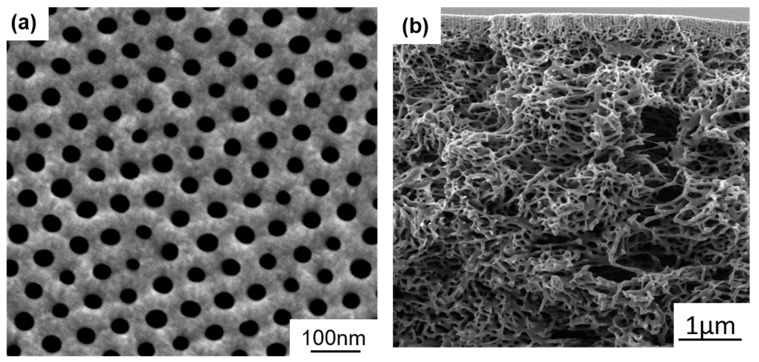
SEM images of (a) top view and (b) cross-section of PS_71_-*b*-P4VP_26_-*b*-PSMA_3_^145^ membranes cast from 23 wt. % solution in a THF/DMF/acetone: 50/30/20 wt. %. Evaporation time was 10 s before immersion in water.
